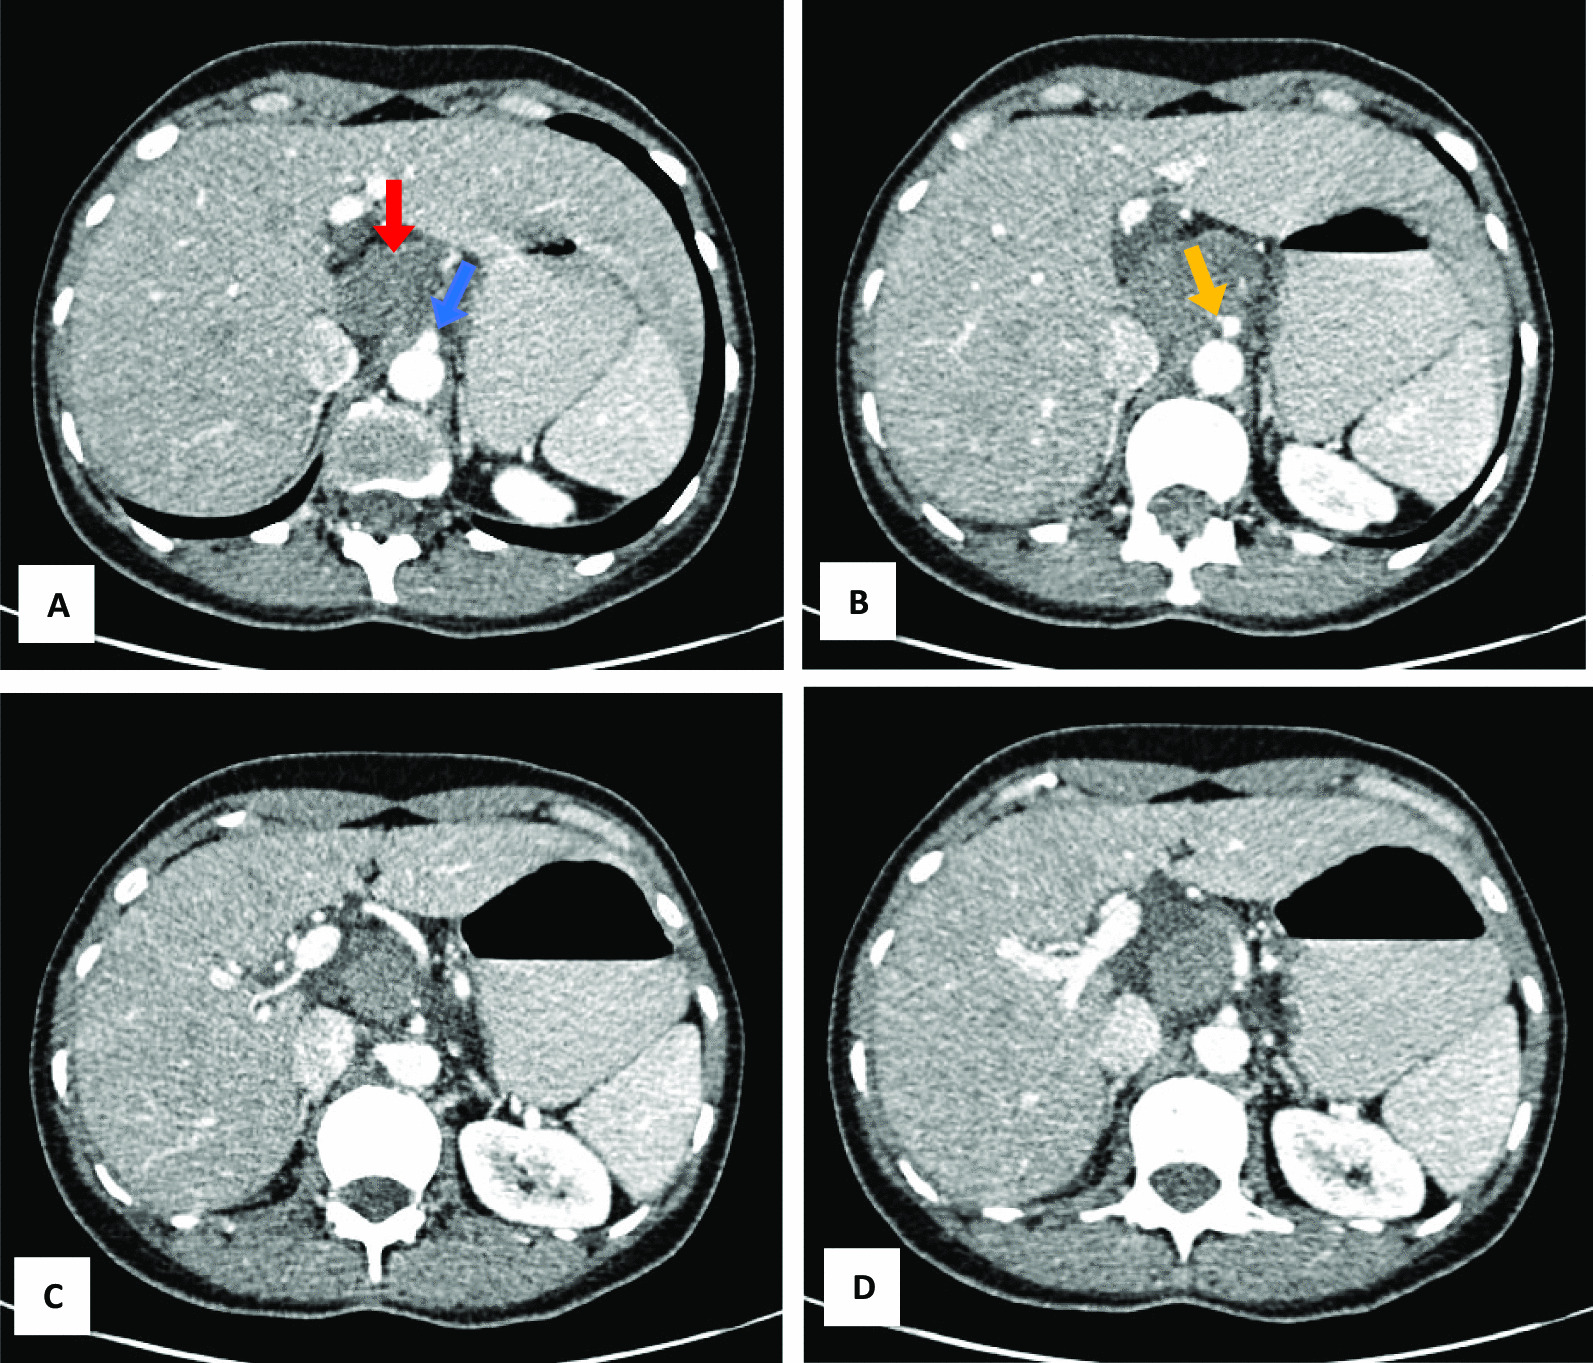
A–D Abdominopelvic CT scan reveals a well-defined hypodense lesion (A marked with red arrow) measuring about 45 × 26 mm^2^ in size, which resembles a soft-tissue mass of unknown origin, without clear enhancement near celiac trunk (A marked with blue arrow), and abutting left gastric artery (B its origin is marked with yellow arrow)
